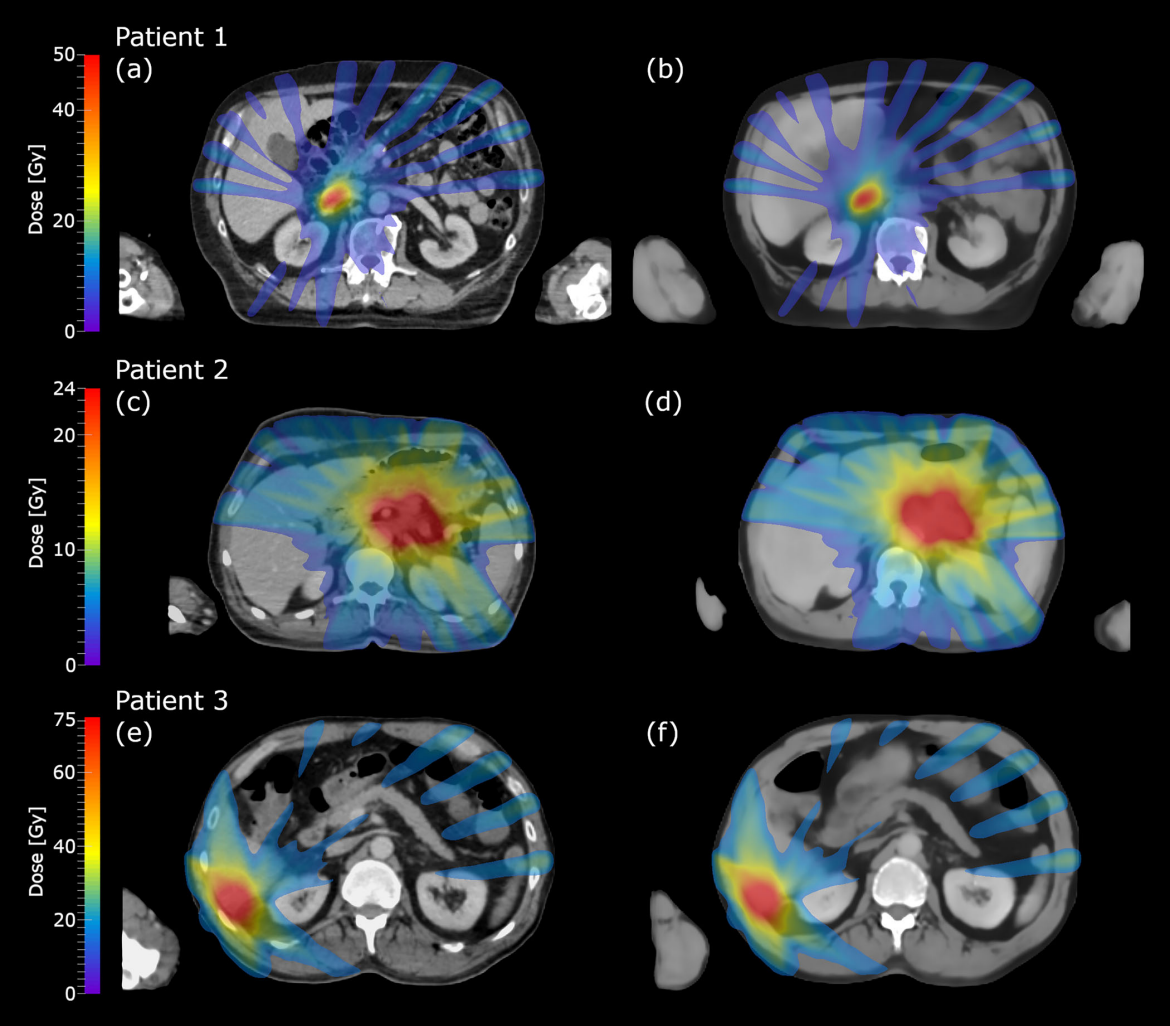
Accumulated dose distributions on deformed computed tomography (dCT) and synthetic CT (sCT). The accumulated physical dose distributions calculated for three patients on dCT (a, c, and e) and sCT (b, d, and f). The prescribed doses are 50 Gy in five fractions to adrenal gland for patient 1, 24 Gy in three fractions to pancreas for patient 2, and 75 Gy in five fractions to liver for patient 3. The dose distributions are thresholded at 5, 3, and 15 Gy for the patients 1–3, respectively. The overall dose differences were among the best for patients 1 and 2, and among the worst for patient 3.
